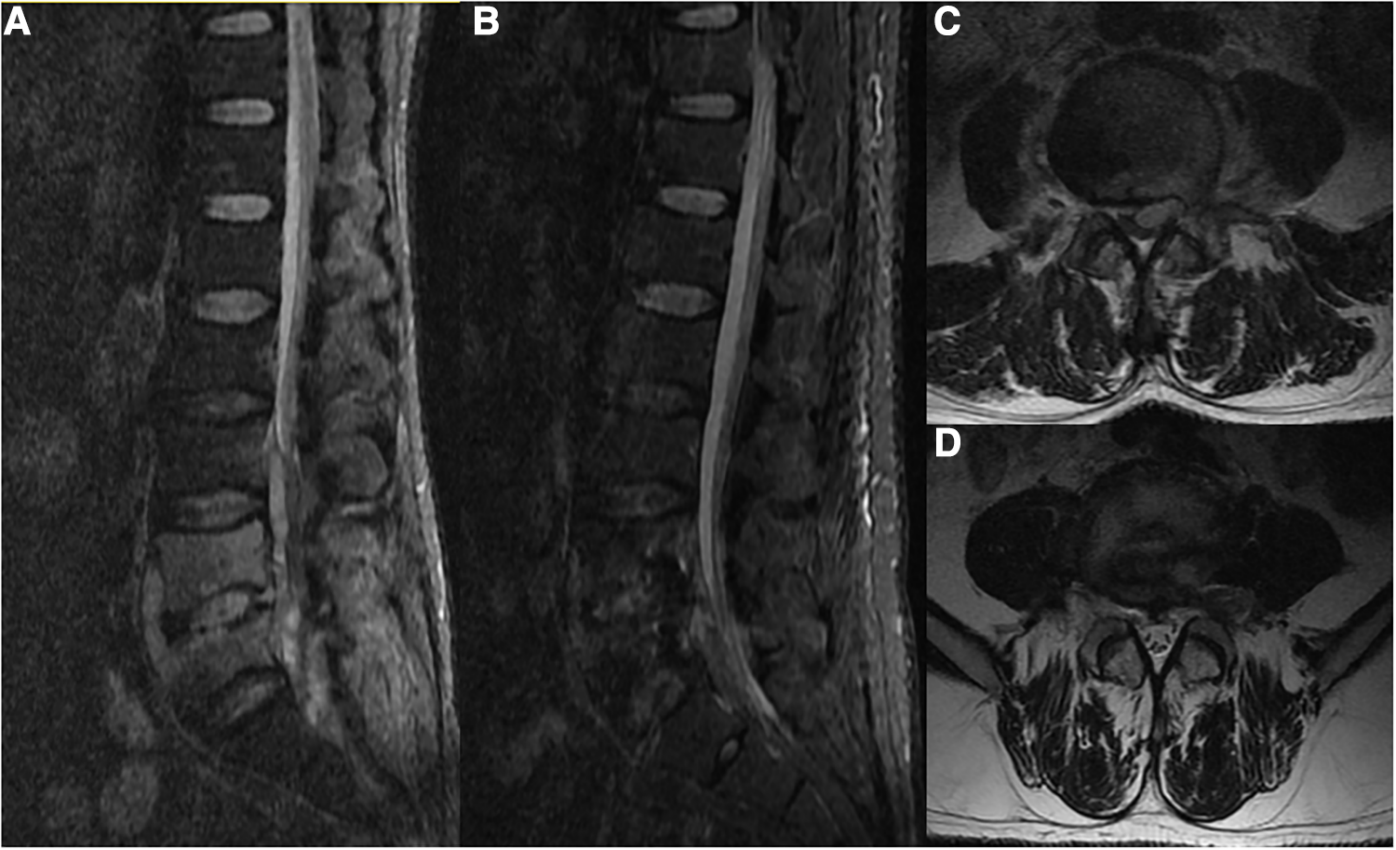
A 40-year-old male presented with Brucella infection of the lumbar spine. (A,C) MRI showed low T1 and high and low T2 mixed signals in the L4, L5 vertebral bodies and the L4/5 space, and patchy high and low mixed signals in the spinal canal behind the L5 vertebral body. The space-occupying lesion was located ventral to the left of the spinal cord, which was considered to be a spinal canal abscess. (B,D) Reexamination of the lumbar MRI after drainage showed that the signal of the L4 and L5 vertebral bodies was significantly improved, and the space-occupying signal in the spinal canal had disappeared.
